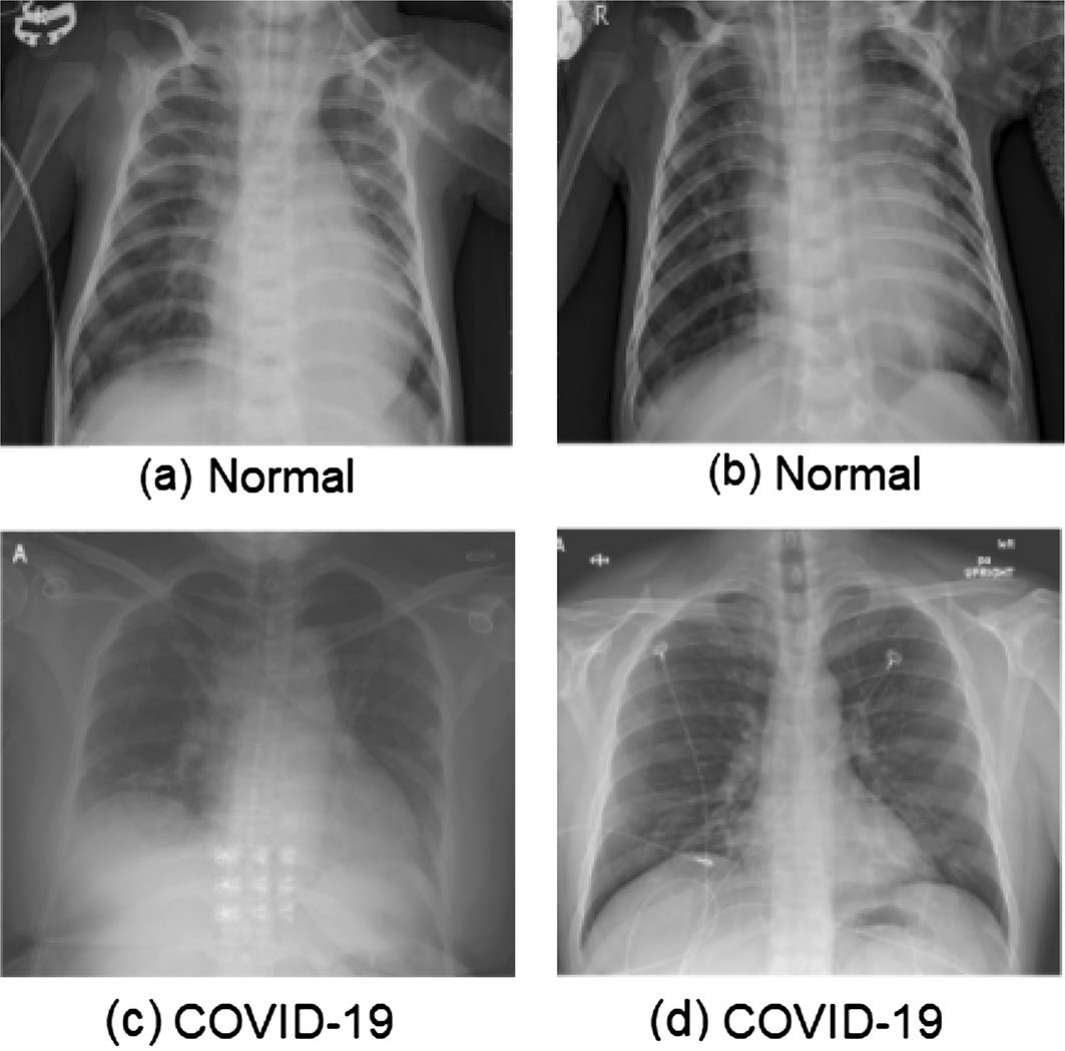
X-ray image samples of COVID-19-infected and healthy (*i.e.*, normal) patients
